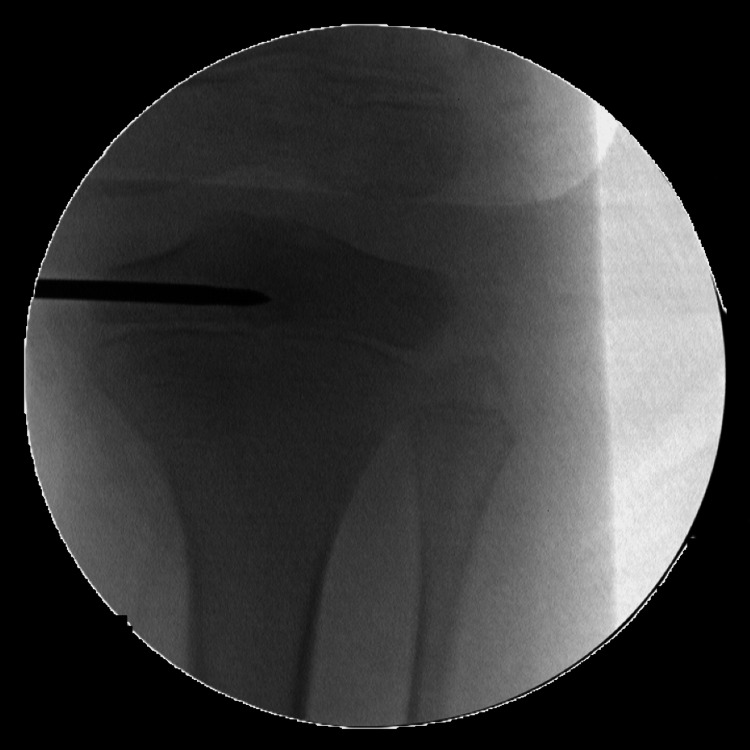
anteroposterior intraoperative radiograph showing the guide wire tip located at the lesion (first surgery)
